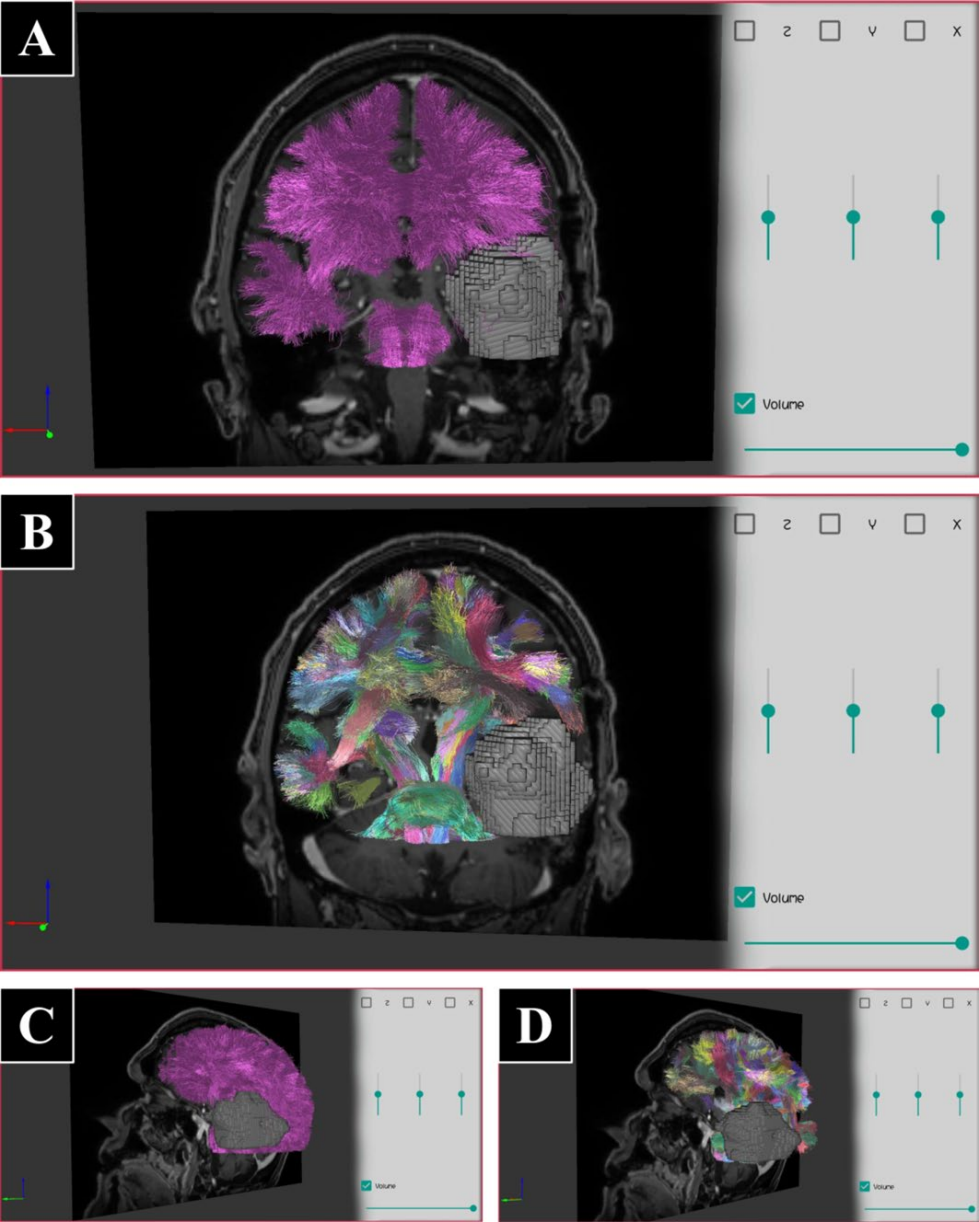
Example of brain data of a patient with a tumor, consisting of a brain MRI and a whole-brain tractography dataset with 71,361 fibers (Dataset III). Also, the dataset contains a brain mask of the segmented tumor which is displayed using volume rendering (VR), with maximum alpha, and a tractography dataset containing the 314 larger fiber clusters. A A coronal slice of the brain image, the whole-brain tractography displayed in purple, and the VR of the tumor. B A coronal slice of the brain, the 314 clusters displayed with random colors, and the VR of the tumor. C, D The same as A and B, respectively, but with a sagittal brain slice (sagittal oblique view)
